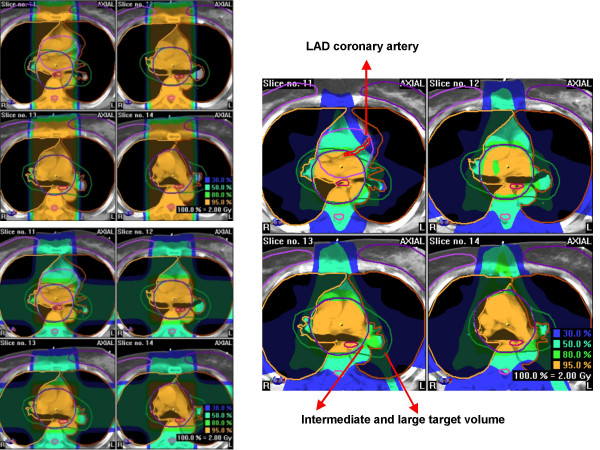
Treatment planning computed tomography scan with contoured organs at risk (incl. left anterior descending coronary artery in red color, on the small images in green color), clinical target volume (both intermediate and large scenario in the same patient) and isodose distributions for the intermediate scenario with ap-pa (upper left), 4-field (lower left), and 7-field IMRT technique (right) in the same patient.
